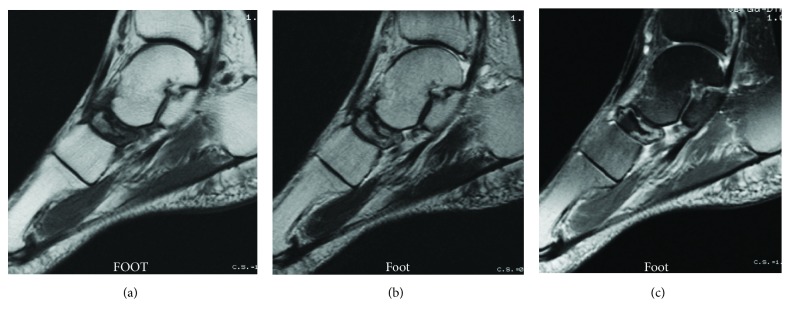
Sagittal MRI shows low signal-intensity areas on both T1-weighted images (a) and T2-weighted images (b) in the marrow of the tarsal navicular. Gd-based MRI (c) shows increased uptake in the peripheral tarsal navicular.
